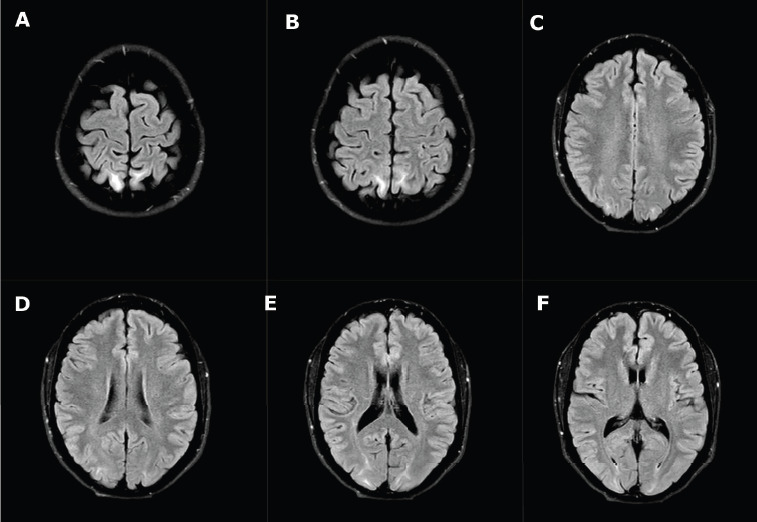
MRI findings revealed FLAIR hyperintense signals in the parieto-occipital subcortical regions, consistent with posterior reversible encephalopathy syndrome (A–F)
